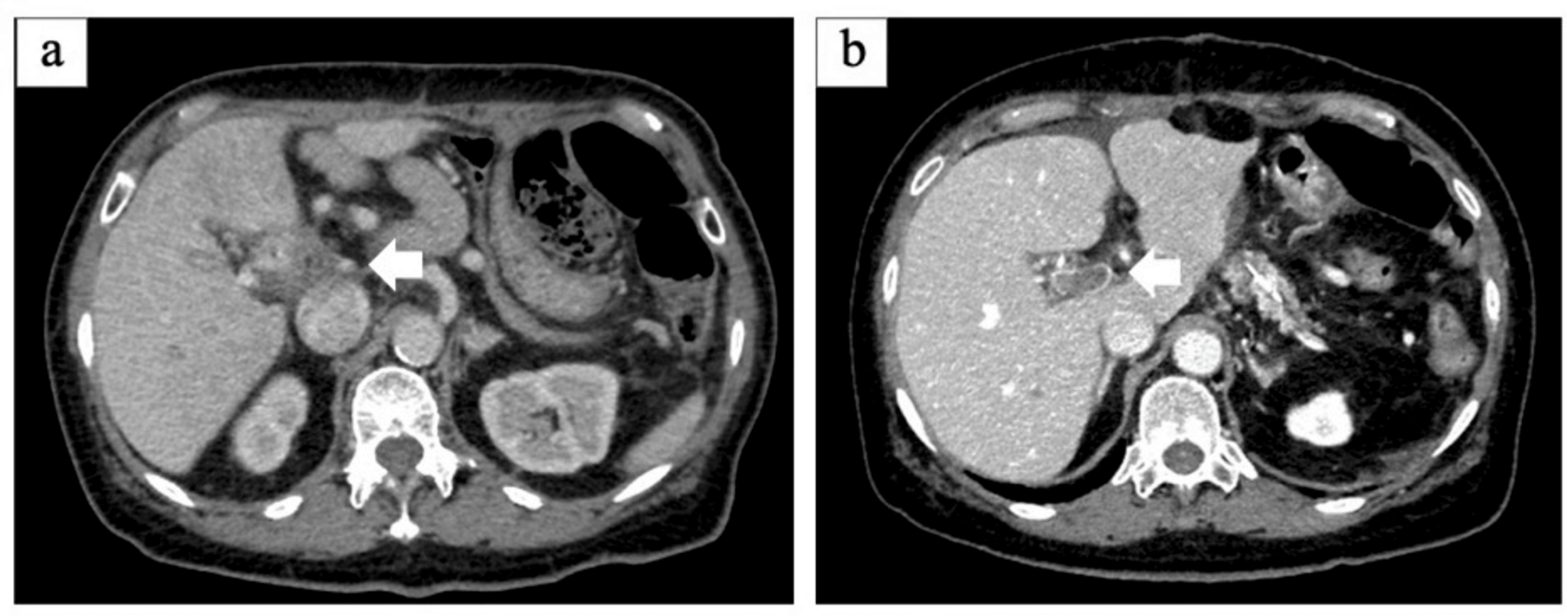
Postoperative one-week axial CT images (a) Portal vein stenosis (arrow); (b) Portal vein thrombus (arrow)
